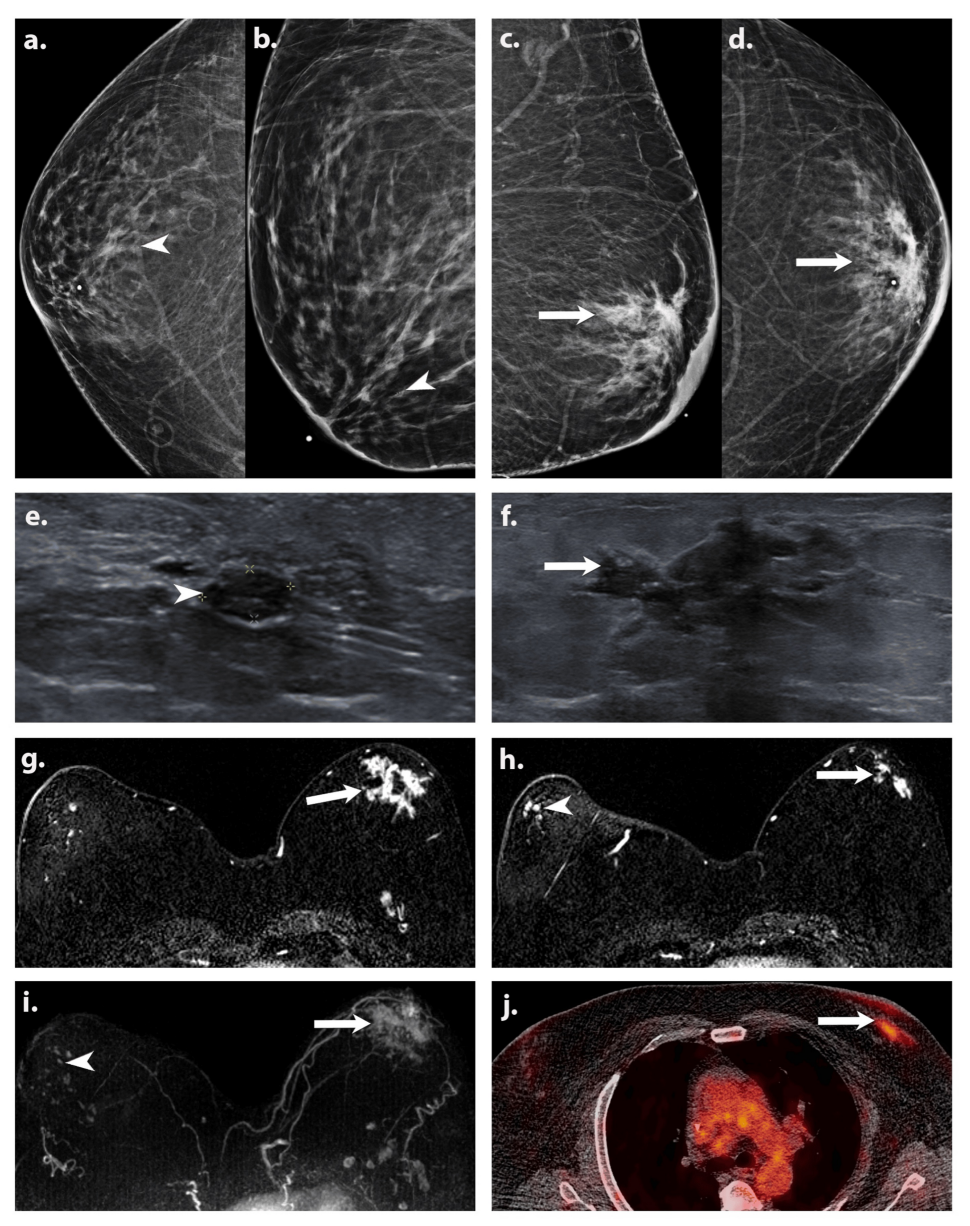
A 74-year-old African American male presented with a palpable left breast mass. Cranio-caudal (CC) and mediolateral oblique (MLO) views of a diagnostic mammogram (a-d) show suspicious microcalcifications within the retroareolar right breast and an irregular spiculated mass within the anterior left breast (white arrows) with overlying skin thickening. Targeted greyscale ultrasound (e) of the right retroareolar breast shows a solid hypoechoic mass (white arrowhead) at 11:00 o’clock, 1 cm from the nipple. Targeted greyscale ultrasound (f) image of the left retroareolar breast demonstrates an irregular spiculated hypoechoic mass (white arrow) from 11:00 to 1:00 o’clock, 3 cm from the nipple. Bilateral ultrasound-guided biopsy revealed left invasive ductal carcinoma (IDC), with associated high-grade ductal carcinoma in situ (DCIS) and right low-grade DCIS. Axial postcontrast T1-weighted fat sat (g,h) and MIP (i) images of the MRI show clumped non-mass enhancement within the left retroareolar breast (white arrows) with associated skin thickening. Segmental non-mass enhancement (white arrowhead) was noted in the right retroareolar region. Axial fused PET/CT image (j) shows hypermetabolic (SUVmax 4.53) left breast mass (white arrow) with skin thickening and minimal tracer uptake in the right breast tissue.
